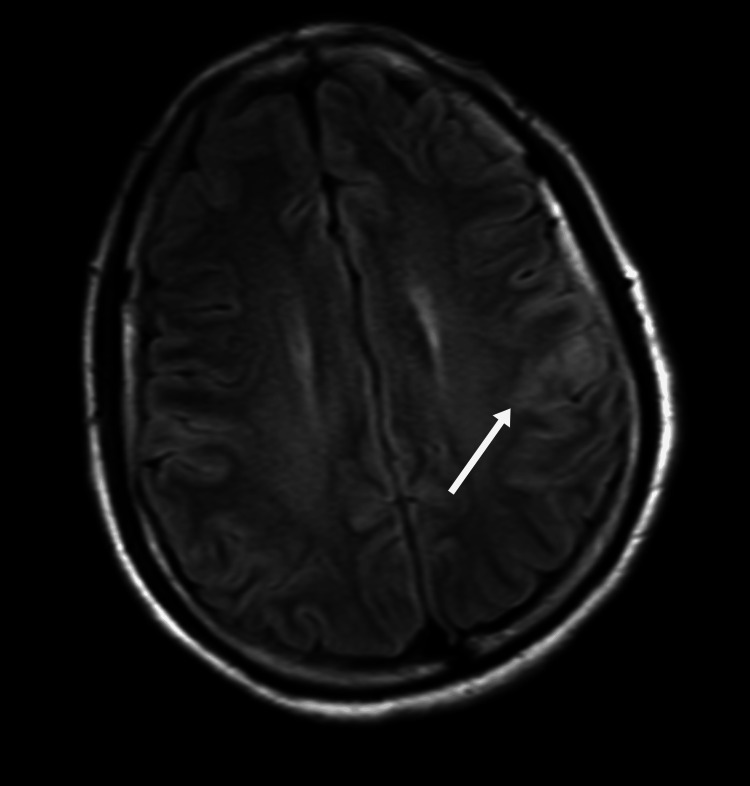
Axial FLAIR MRI showing a hyperintense lesion in the subcortical white matter of the right parietal lobe As indicated by the white arrow, there is a high-signal lesion in the subcortical white matter of the right parietal lobe FLAIR: fluid-attenuated inversion recovery; MRI: magnetic resonance imaging
